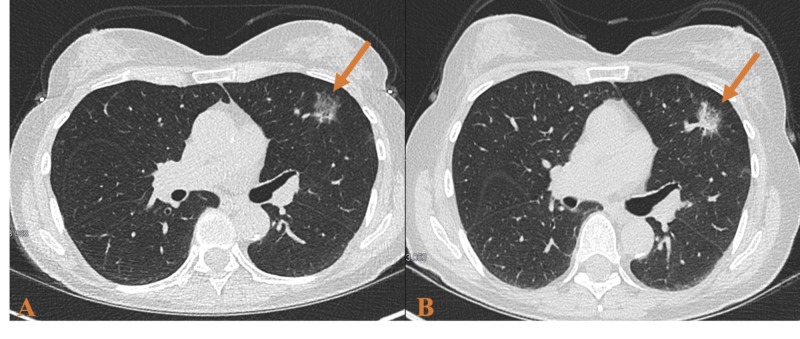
(A) CT scan showing a 2.3 x 1.3 x 0.9 cm ground glass opacity nodule in the anterior left upper lobe. (B) Follow-up CT scan showed the solid nodular component increasing to 2.8 x 1.4 x 1.0 cm.
